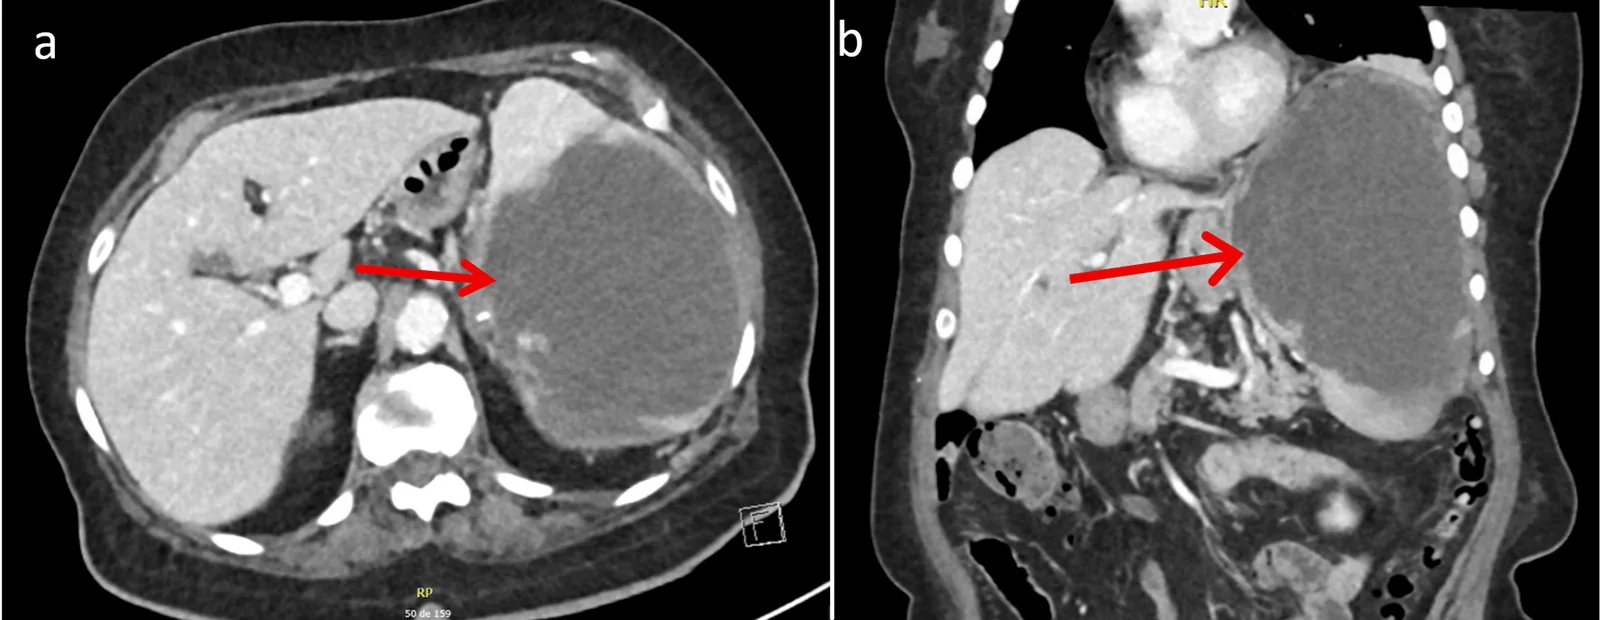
Transversal (a) and coronal (b) sections of the thoraco-abdominal CT scan in portal phase; splenic abscess indicated by arrows
